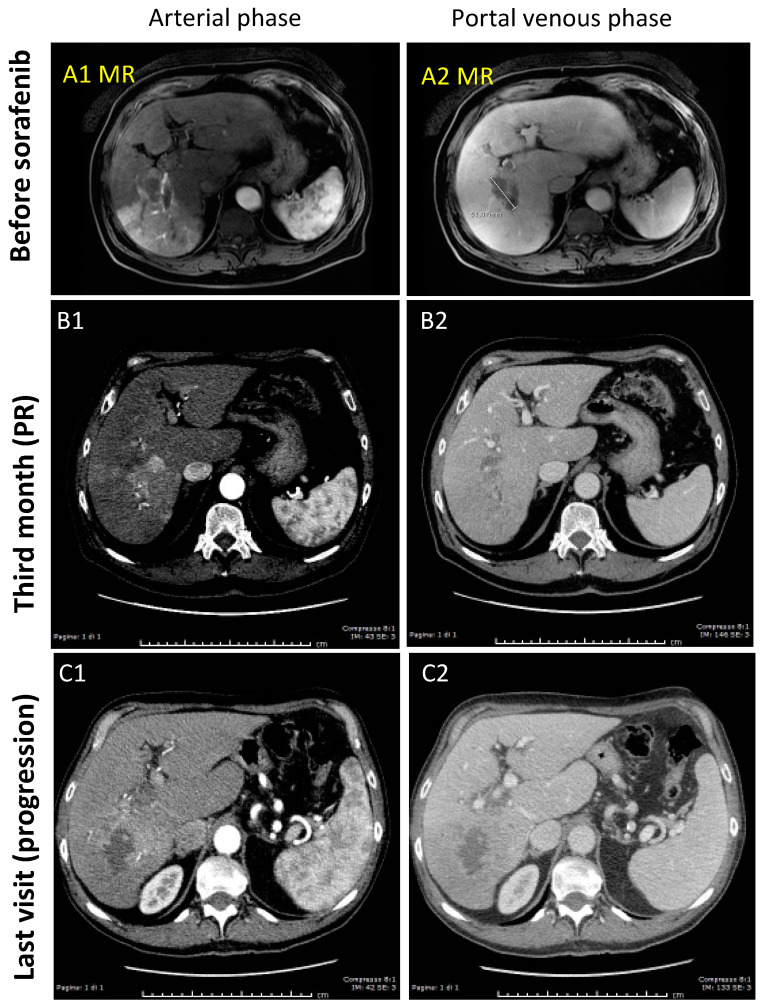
Baseline MRI (A1,A2) showing a widespread hypo-vascular HCC in liver segment IV, with complete thrombosis of the portal branch for the VII–VI liver segments. A partial response after 3 months of sorafenib therapy was documented by CT scans (B1,B2), which has been progressively lost, as shown at the last CT scan performed after 20 months of therapy (C1,C2).
